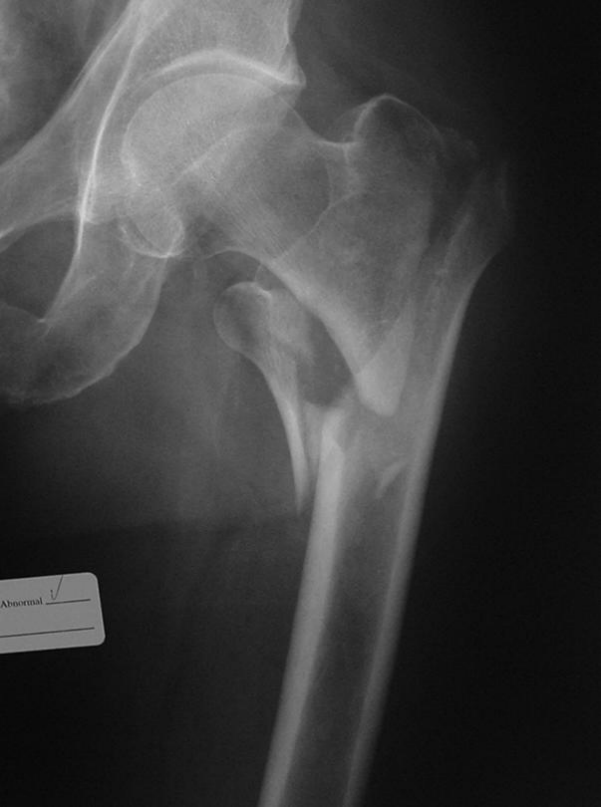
Antero-posterior x-ray of unstable pertrochanteric fracture sustained after simple fall by an elderly patient. Eighteen hours after the injury he started developing signs of acute thigh compartment syndrome and was taken urgently to operating room for anterior thigh compartment fasciotomy. Muscles were still valuable and fracture fixation with cephalomedullary nail was done immediately after. Patient did not have any history of hemorrhagic diathesis, was not taking any blood thinners and there were no abnormalities on patient routine preoperative workup (PT, PTT, INR, platelets). He recuperated well.
